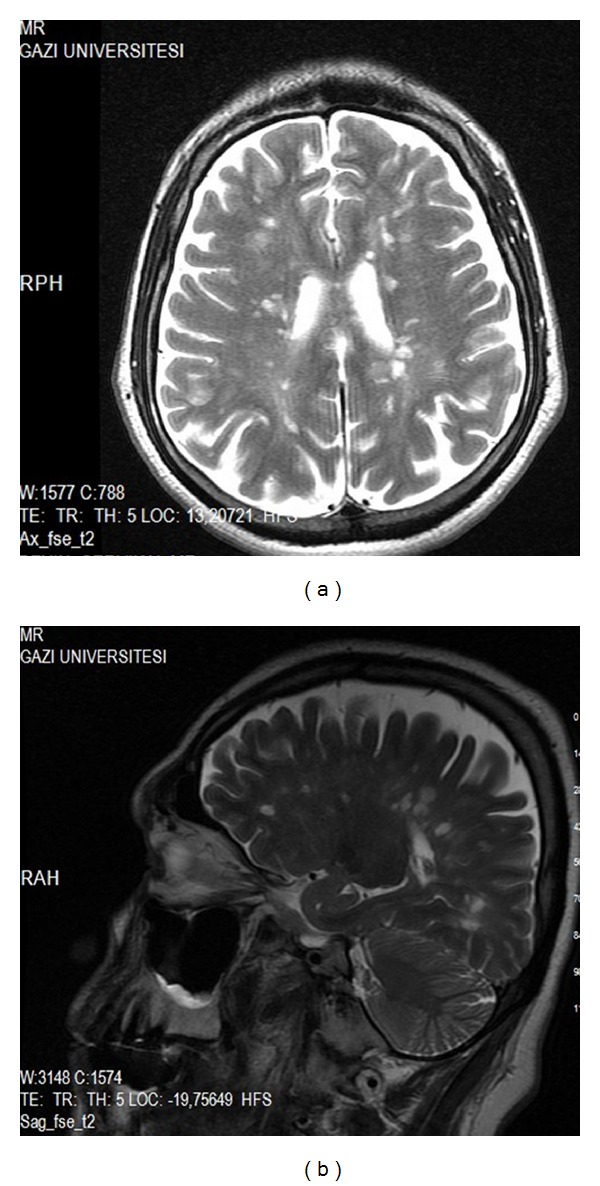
(a) Demyelinating periventricular lesions, T2 weighted axial section, (b) demyelinating periventricular lesions, T2 weighted sagittal section, and control brain MRI.
